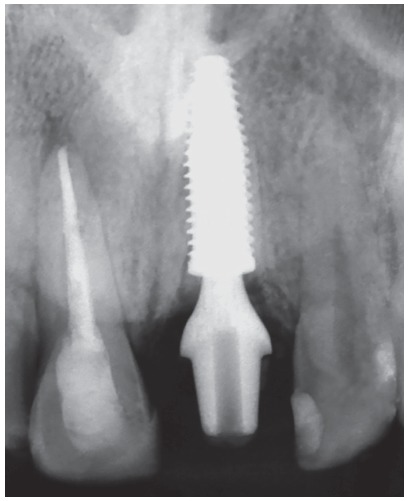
Final radiography of the site after installing the implant in the decoronated central incisor region with replacement resorption. The adjacent central incisor also suffered trauma, but without progression to dentoalveolar ankylosis, and evolved to aseptic pulp necrosis, endodontically treated.
